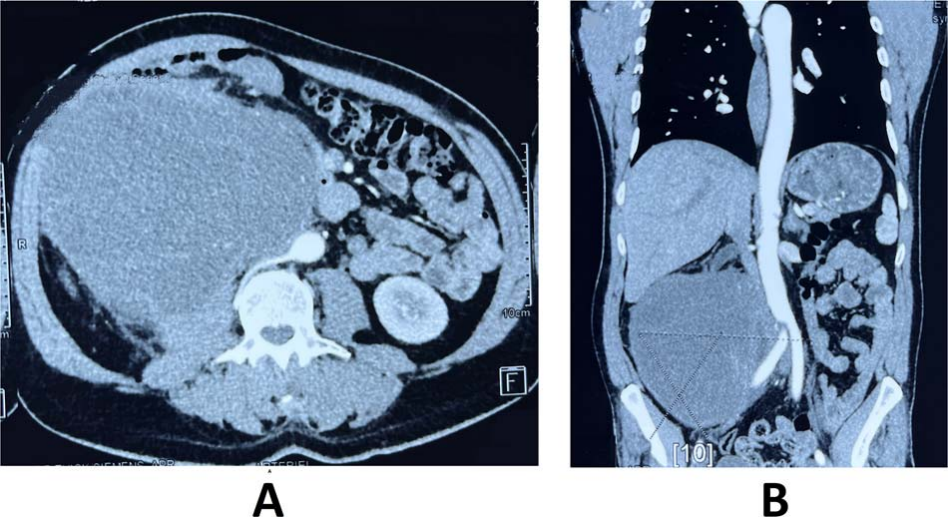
Axial (A) and frontal (B) view of Computed tomography scan showing a large right retroperitoneal solid mass, with lobulated thickened wall measuring 190 × 140 × 120 mm, heterogeneously enhancing, displacing anteriorly duodenal loops, laterally the right colon, medially the inferior vena cava and abdominal aorta.
